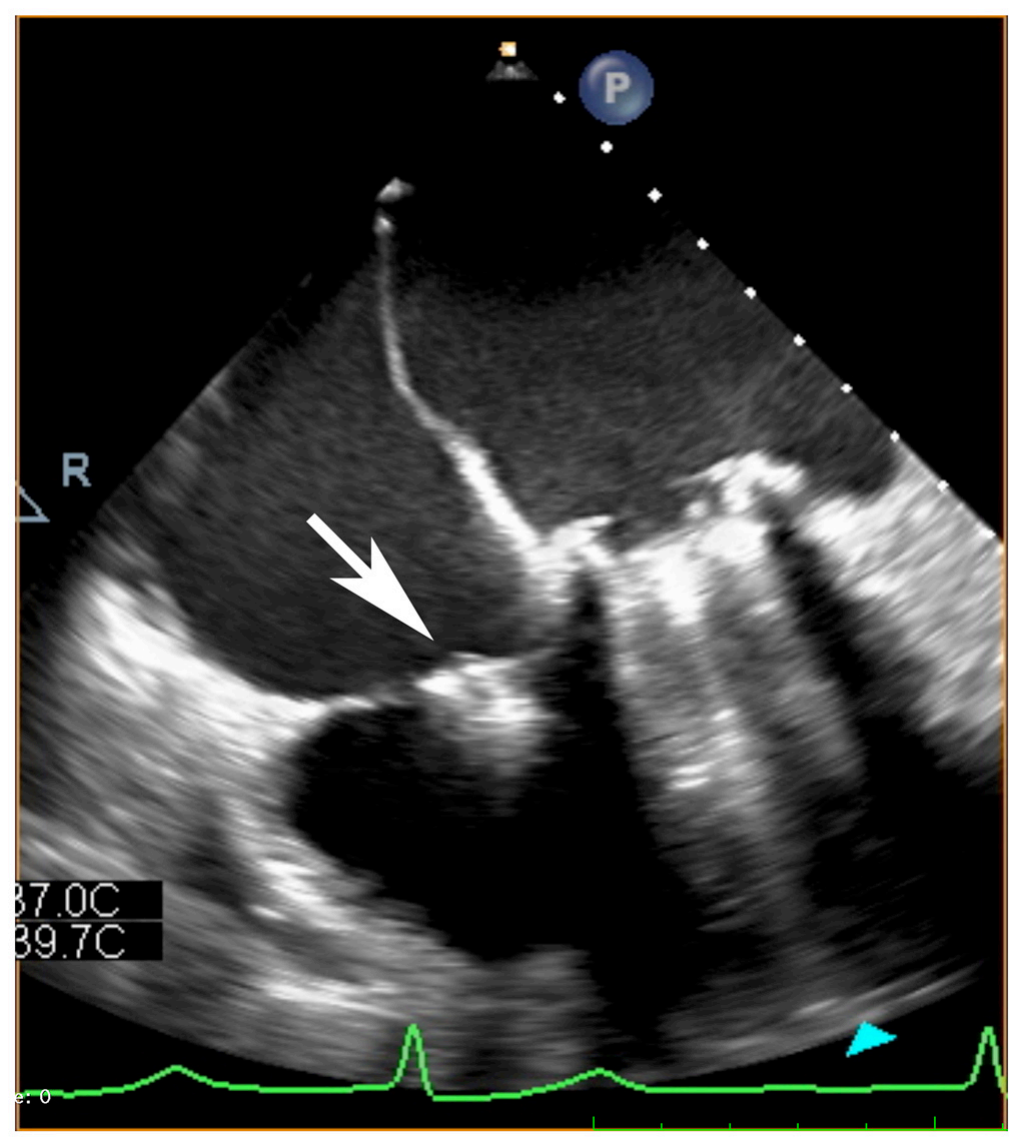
Trans-esophageal echocardiographic image showing a TriClip device.
